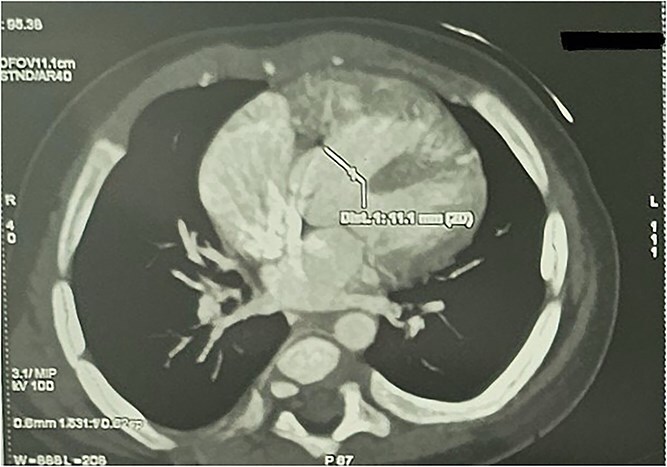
CTA demonstrating malaligned perimembranous ventricular septal defect with ToF morphology.
